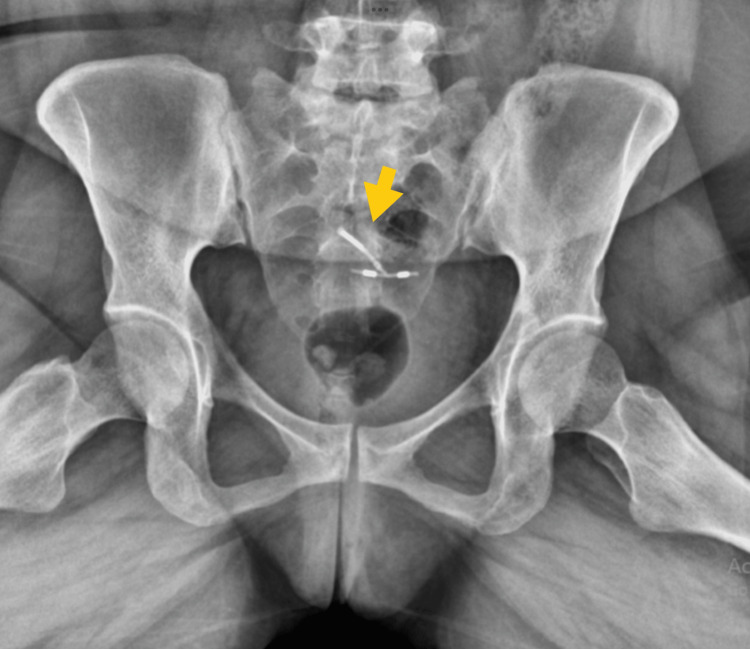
Pelvic X-ray demonstrating an anteroposterior (AP) view of the IUD in the pelvic cavity (yellow arrow). IUD: Intrauterine device.
